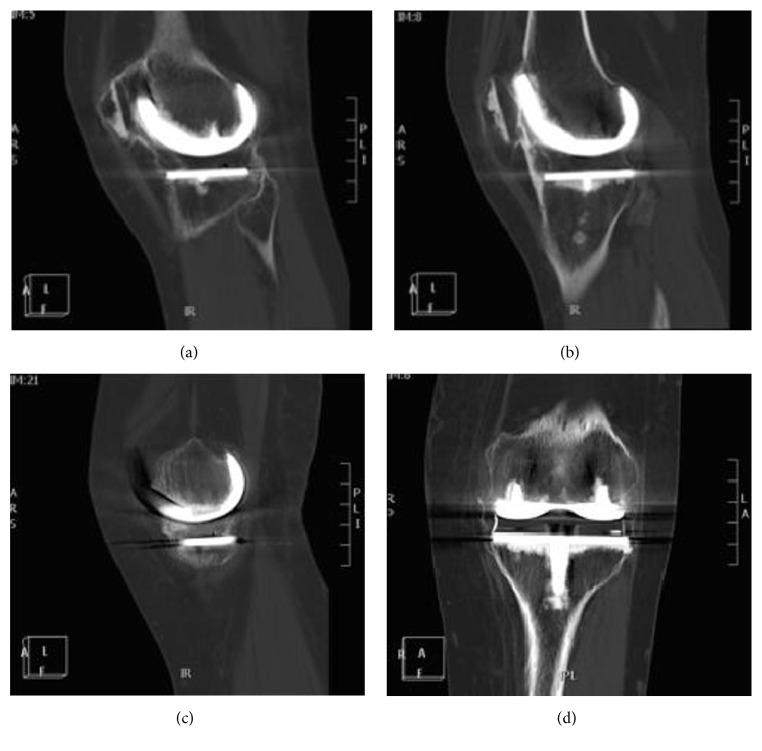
CT scan views showing a circumferential complete ankylosis of the knee.
